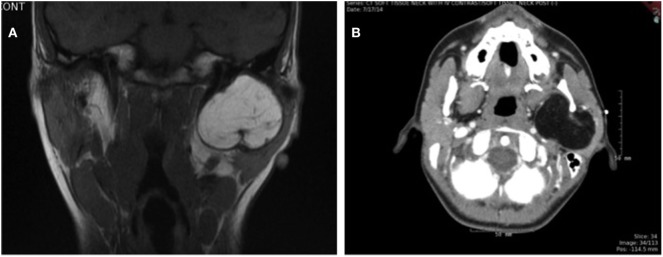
(A) T1-weighted coronal MRI image and (B) axial CT image showing large mass in left parotid gland.
